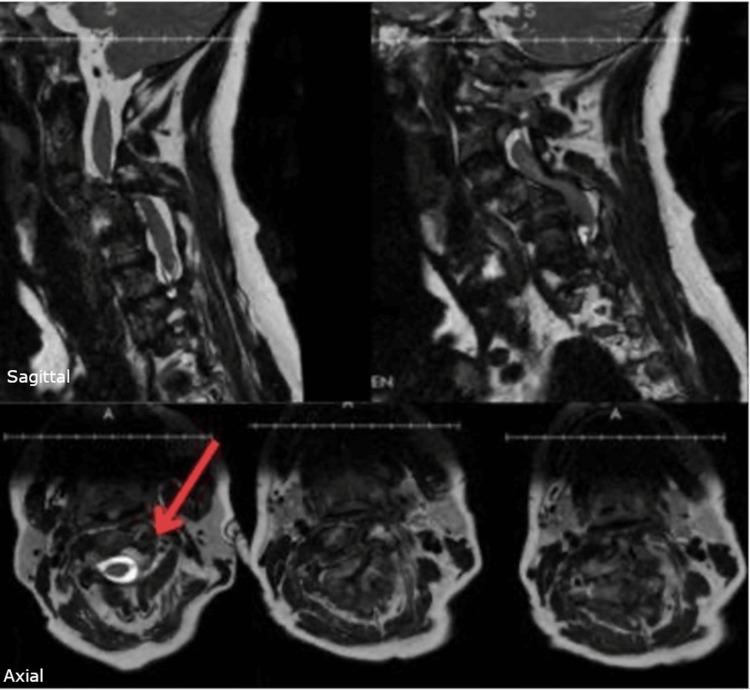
Preoperative T2-weighted Sagittal and Axial Cervical MRI Preoperative T2-weighted cervical MRI demonstrating severe multilevel spinal canal stenosis from C4-6 at the apex of cervical kyphosis and atypical vertebral artery anatomy on left at C2 (arrow).
